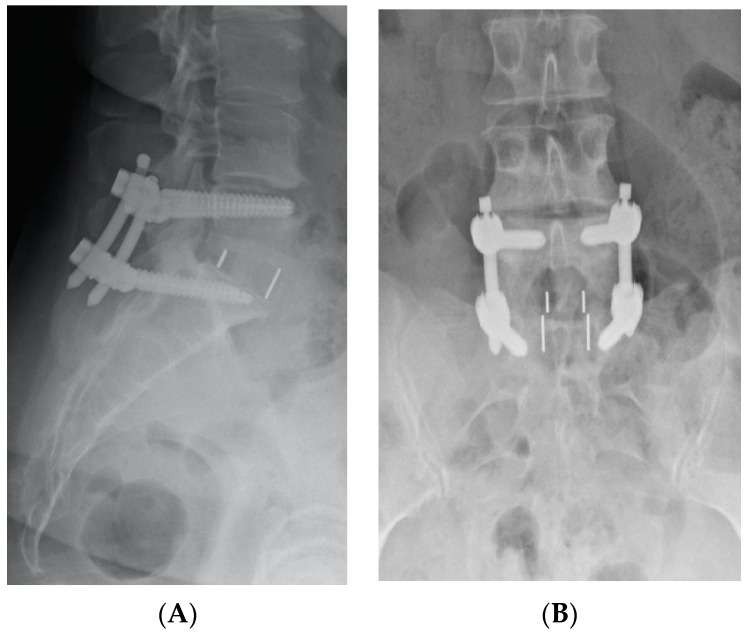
X-ray images: (A) lateral view and (B) coronal view of an ALIF procedure with a cage in the L5/S1 segment and dorsal fixation with pedicle screws.
